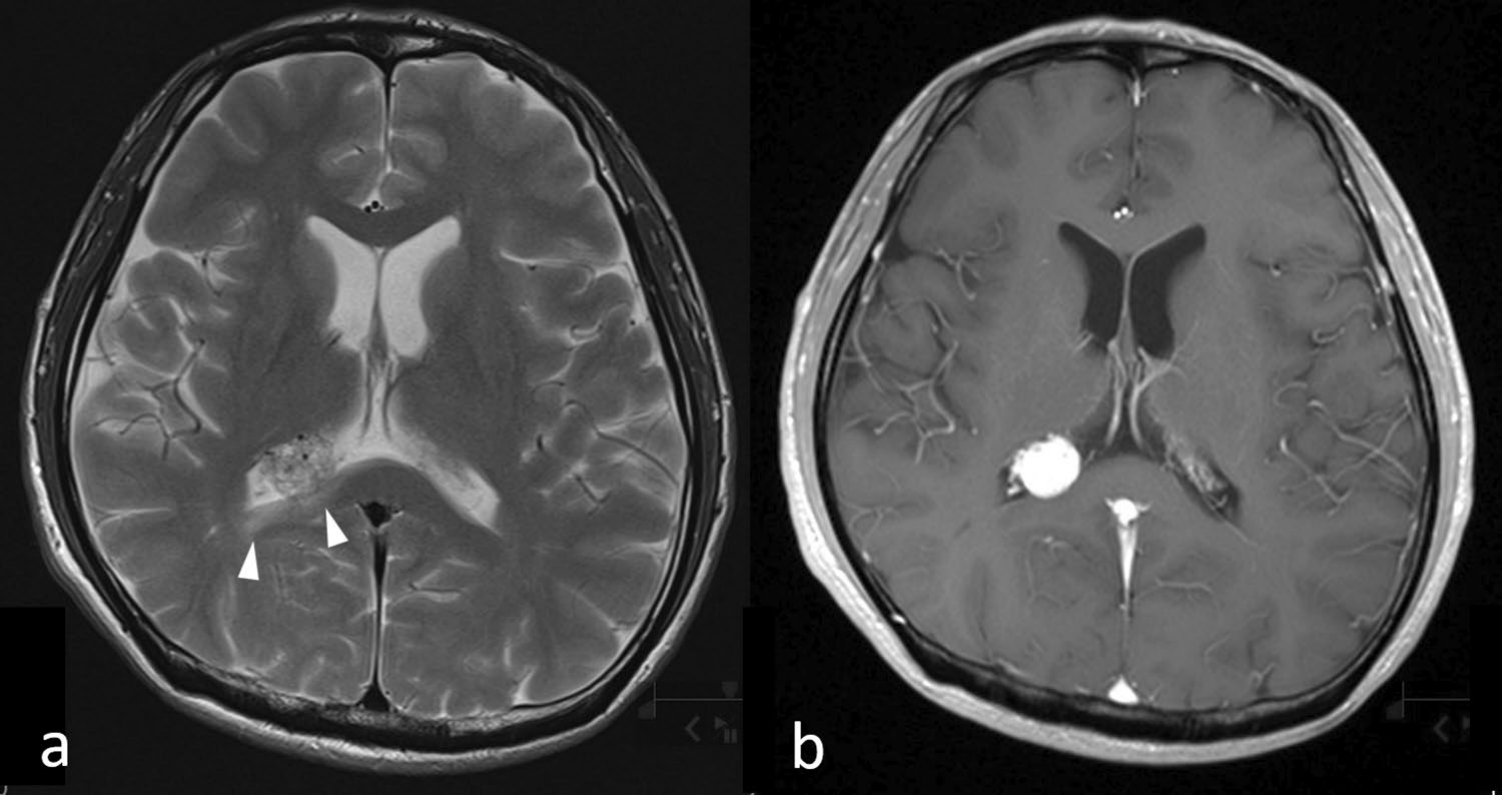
Choroid plexus metastasis in a patient with renal cell carcinoma. T2-weighted image a shows a moderate hyperintense tumor in the right lateral ventricle with surrounding hyperintense area (arrow heads) corresponding to edema in the right part of the splenium of the corpus callosum. A contrast-enhanced T1-weighted image b shows the tumor with homogeneous intense enhancement
